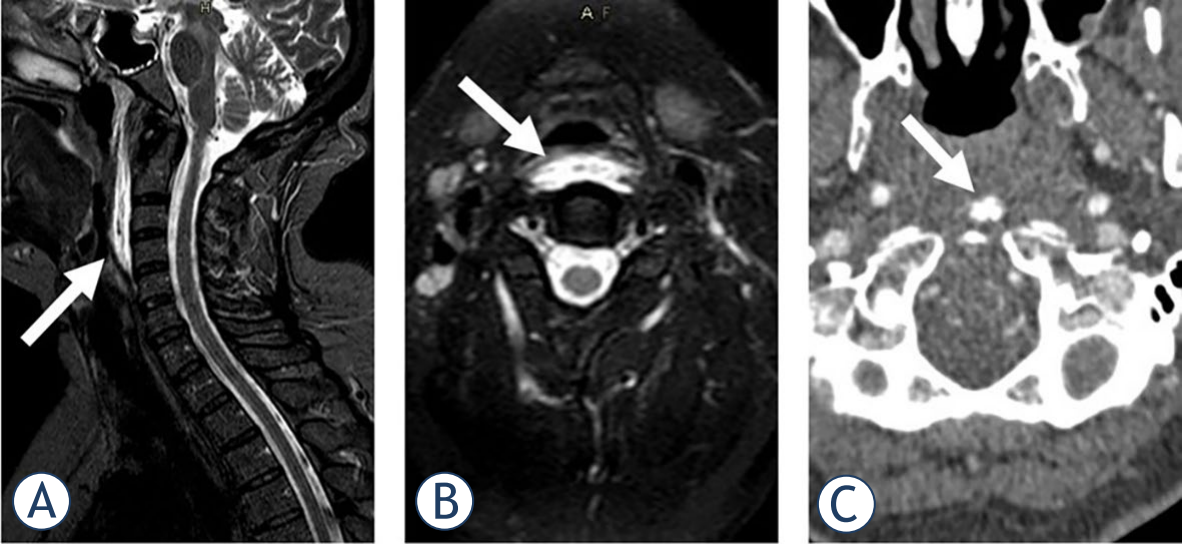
STIR (short tau inversion recovery) imaging in sagittal (A) and axial (B) plane demonstrated prevertebral soft tissue swelling and oedema in a 51-year-old female, suggesting retropharyngeal calcific tendinitis as the underlying cause. Calcifications in the medial aspect of the longus colli muscle in front of the C1 arc were noted on computed tomography angiography (CTA) (C), confirming the diagnosis.
